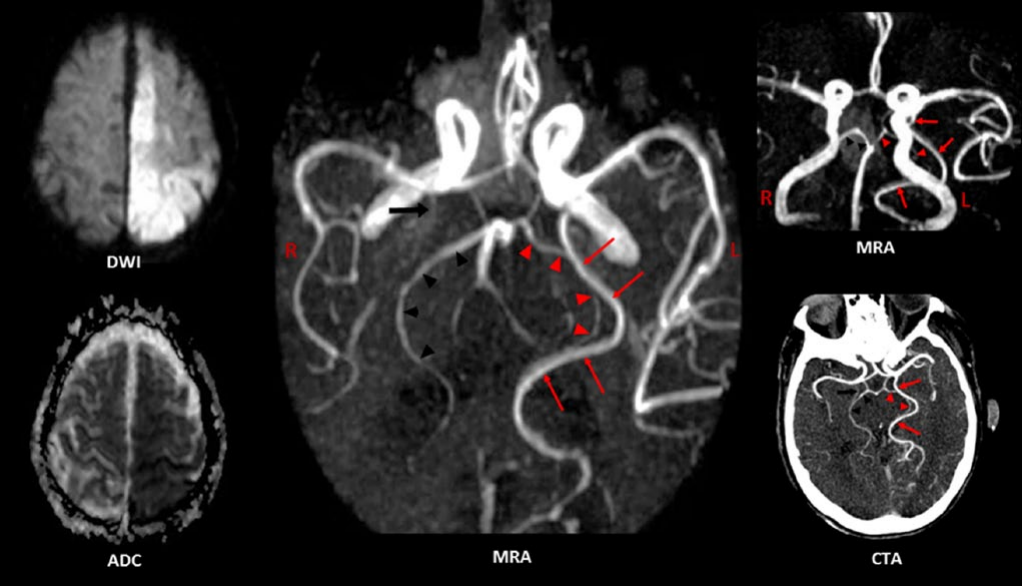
Patient 2 had acute infarcts in the left ACA, MCA, and PCA territories (hyperintense DWI, low ADC). The 3D time-of-flight (TOF) MRA images show 2 PCAs on the left (PCA duplication)—a dominant fetal-type PCA (arrows) and a small-caliber classic PCA (arrowheads). The left PCA and the right PCA (dark arrowheads) branch off the basilar artery. Two posterior communicating arteries and a right anterior choroidal artery (large dark arrow) are also present (middle MRA and CTA images). There is no evidence of flow-disrupting stenosis.
